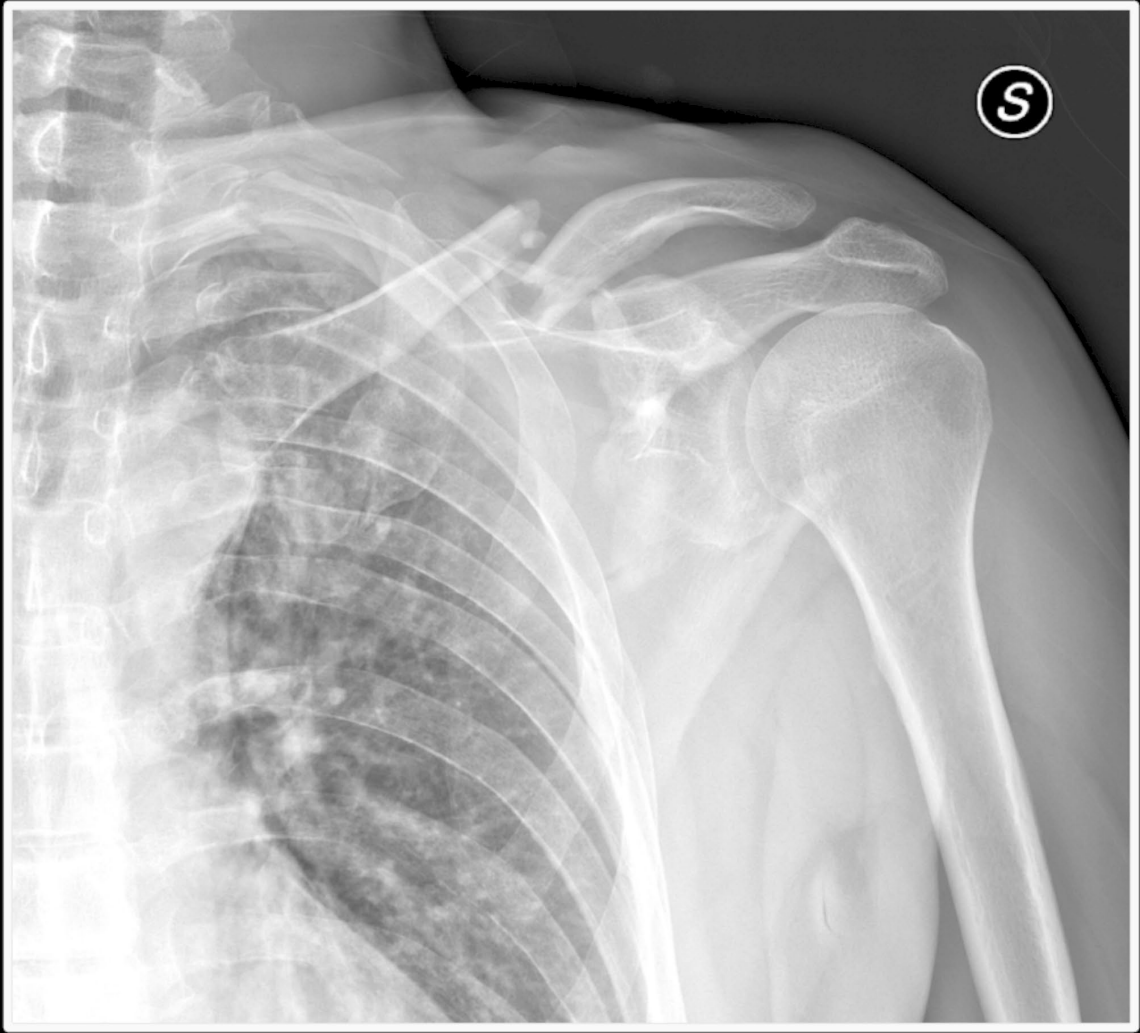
Initial X-ray of the left shoulder in AP view
